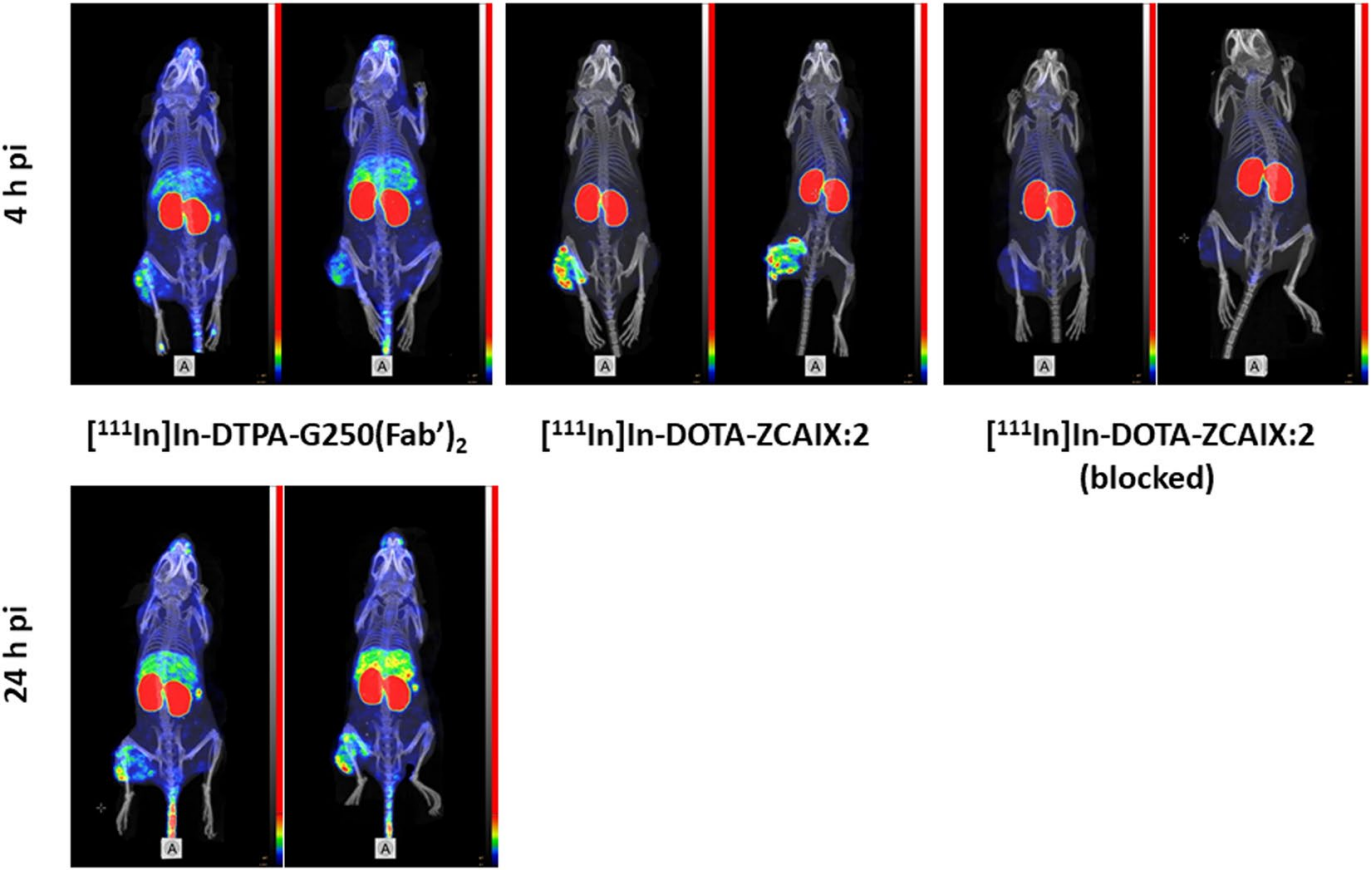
SPECT/CT imaging of CAIX-expressing SK-RC-52 xenografts using [^111^In]In-DOTA-ZCAIX:2 and [^111^In]In-DTPA-G250(Fab’)_2_. Images are presented as maximum intensity projection (MIP) in RGB (red, green and blue) color scale.
